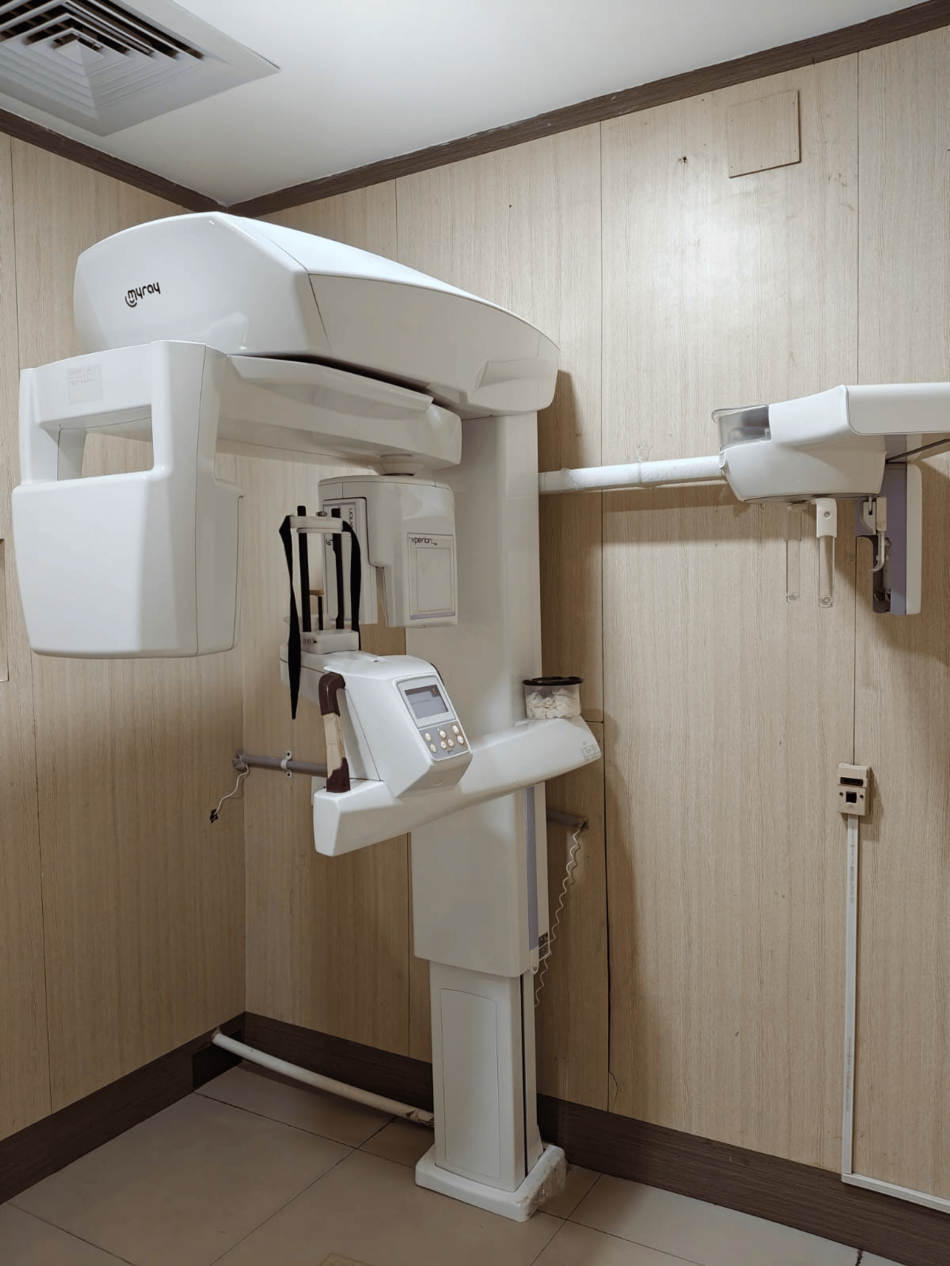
Hyperion X9 CBCT Scanner (MyRay, Charlotte, NC, US), Kalinga Institute of Dental Sciences, Kalinga Institute of Industrial Technology
